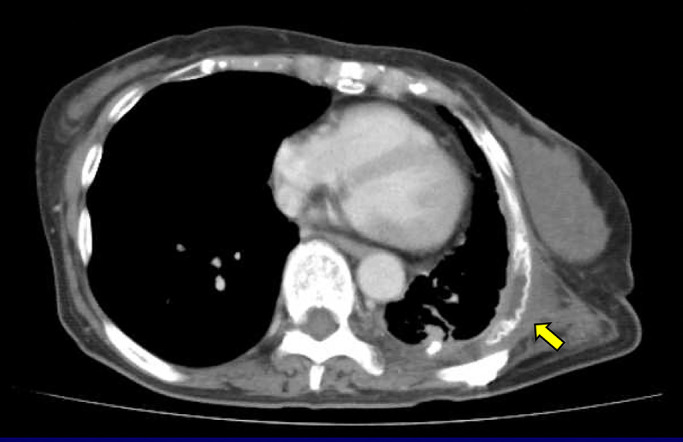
Computed tomography 3 months after the reconstruction showing good lung expansion with no atelectasis despite seroma formation. The yellow arrow indicates the Dual Mesh.
